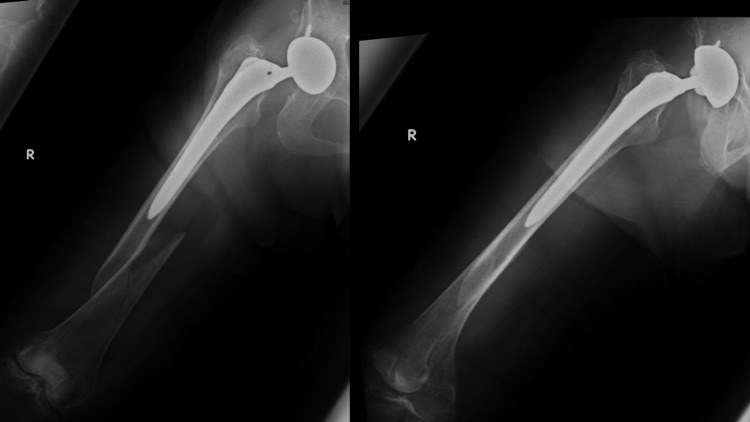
Preoperative radiographs of case 4 Preoperative radiographs of case 4 showed PFF of Vancouver classification Type C1. PFF: periprosthetic femoral fracture.
